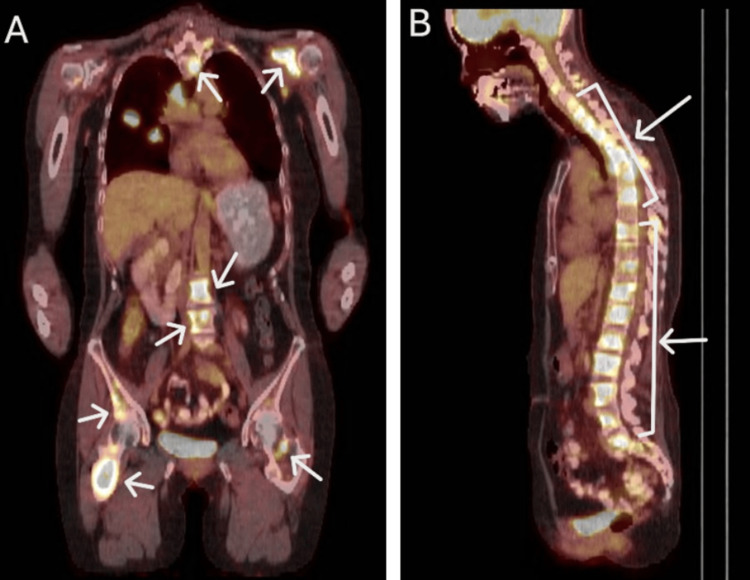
Sagittal (Panel A) and coronal (Panel B) views of positron emission tomography/computed tomography (PET/CT) showing diffusely metastatic disease in the bones, liver and lung.
